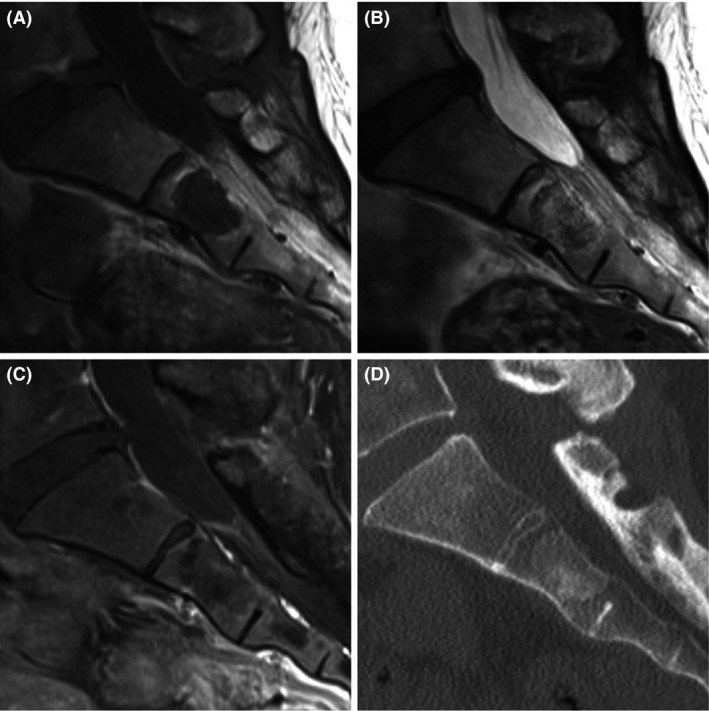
Imaging findings in case 2. On MRI of the sacral spine, the signal intensities of the tumorous lesion are homogeneously low in T1‐weighted images and high in T2‐weighted images, and no enhancement is observed in fat‐suppression T1‐weighted images with a gadolinium contrast medium in the S2 vertebral body (A−C). CT image demonstrates slight osteosclerosis throughout the tumorous lesion and no osteolytic changes (D).
